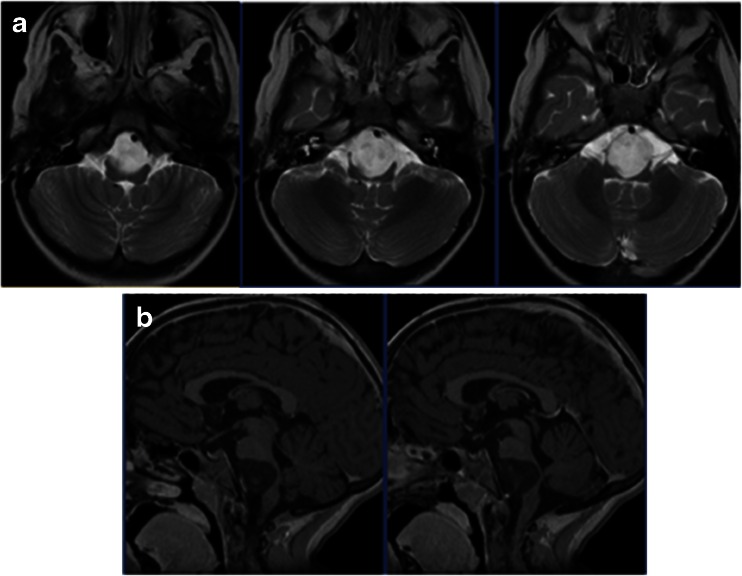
Case 3. Four-year-old girl with previous history of chemical meningitis. MR images (a T2-weighted axial view, b T1-weight sagittal image after contrast infusion) showing a large mass ventral to the ponto-medullary junction. Note a severe brain stem compression by the non-enhancing tumor between the brain stem and the basilar artery
